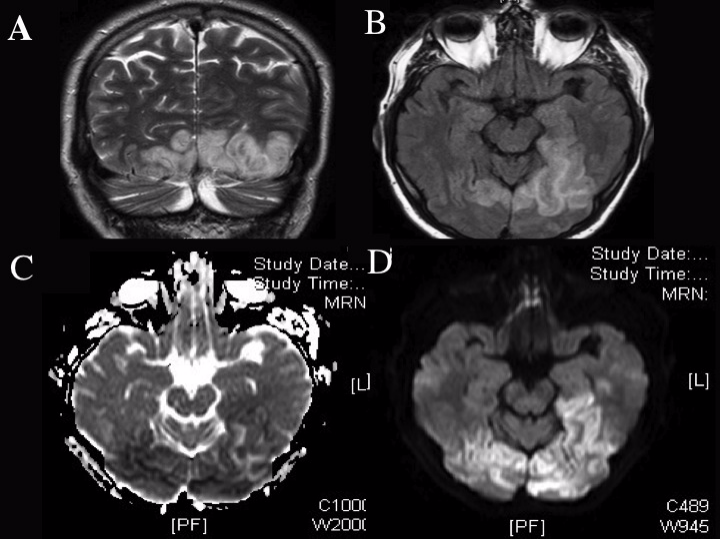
(A) MRI scan coronal view, T2 weighted, (B) axial FLAIR sequence, (C) Acquired diffusion coefficient and (D) Diffusion weighted image, showing area of abnormal signal in both occipital lobes and extending into the inferomedial aspect of the left temporal lobe, consistent with acute cerebral infarction.
